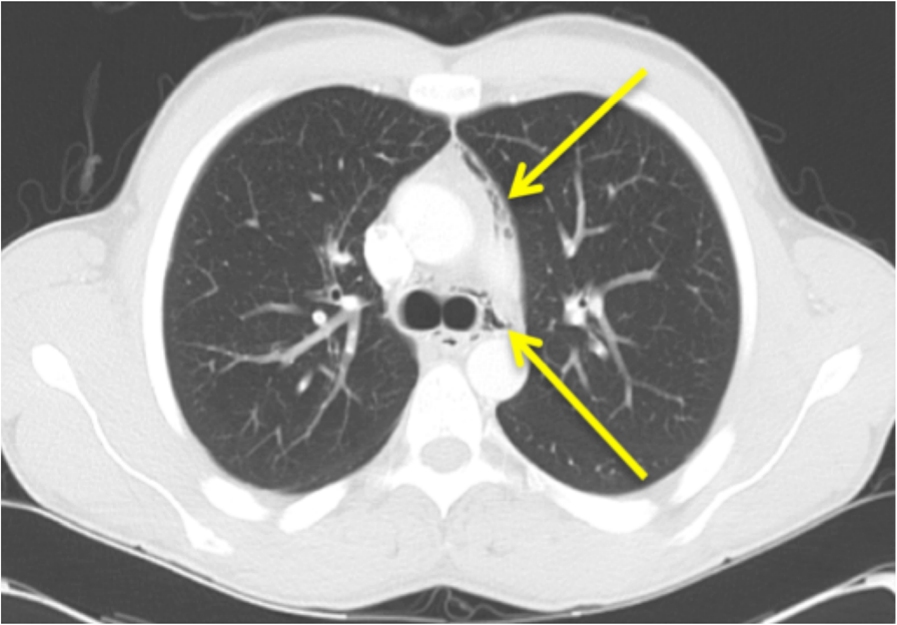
Axial chest computed tomography demonstrating multiple locules of gas within the mediastinum (both arrows)
